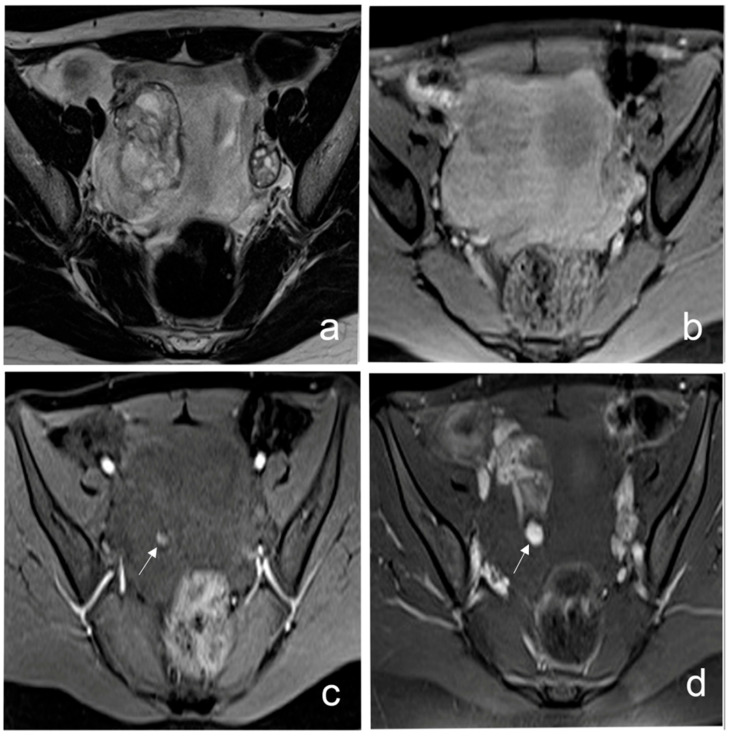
Ruptured hemorrhagic ovarian cyst with hemoperitoneum in a 17-year-old female presenting with acute pelvic pain. (a) Axial T2-weighted image shows a right adnexal mass with surrounding hemorrhagic fluid. (b) Axial T1-weighted image demonstrates hyperintense hemorrhagic content within the cyst. (c,d) Axial contrast-enhanced T1-weighted image reveals active contrast extravasation (arrow) into the peritoneal cavity, confirming ongoing bleeding from the ruptured cyst. These findings established the diagnosis of a ruptured hemorrhagic ovarian cyst with hemoperitoneum.
